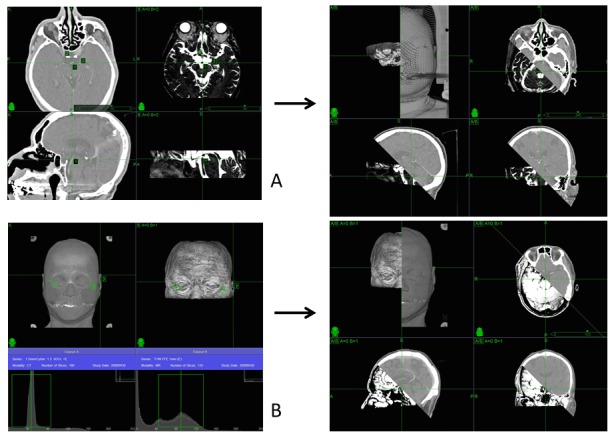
MR to CT image fusion registration. Image fusion of point-based registration (A) and intensity-based registration (B). MR heavy T2-images fused to thin-sliced CT axial sections using point-based and Gd-enhanced MR images using intensity-based registration. Before (left) and after image fusion (right).
